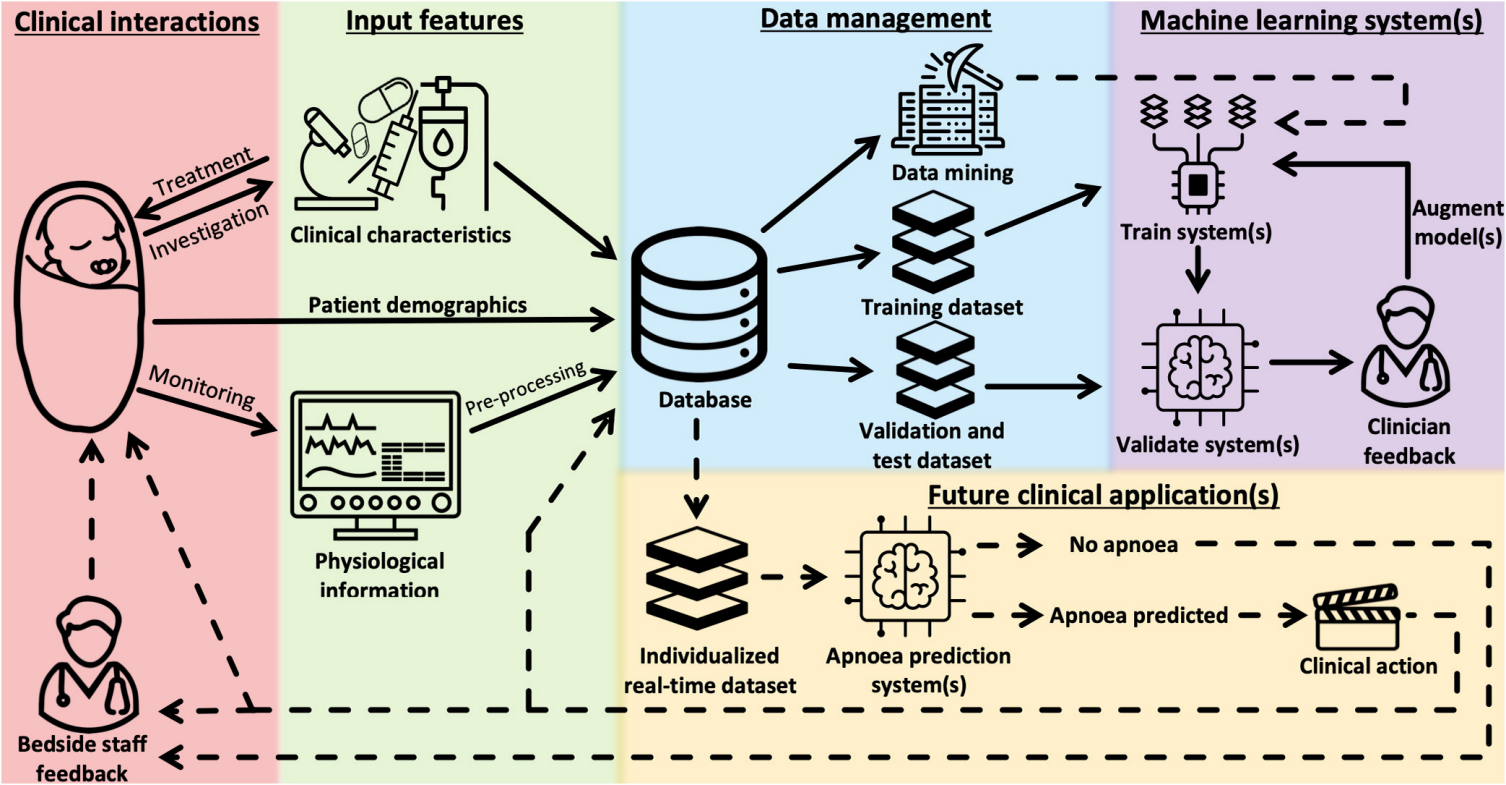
Development of a machine learning-based system for prediction of apnoeic events in preterm infants. Solid lines, Steps already undertaken to develop a predictive system; Dashed lines, Future steps for development and application of a machine learning system to predict apnoeic events. *Patient demographics*: may include birth gestation, postnatal age, birth and current weight, Apgar scores, and medical diagnoses. *Clinical characteristics*: may include mode, duration and level of respiratory support, dosage and timing of previous and current treatment (e.g., surfactant therapy, post-natal corticosteroid, caffeine), and investigation findings (e.g., hemoglobin, radiographic findings). *Physiological information*: may include heart rate and R-R interval, respiratory rate and inter-breath interval, movement patterns, and characteristics of previous apnoeic events (e.g., type and duration of event, latency between events, apnoea-associated destabilization). *Clinical action*: may include an early warning display, a servo-controlled stimulus to maintain respiratory cadence, or decision support regarding further preventative management.
